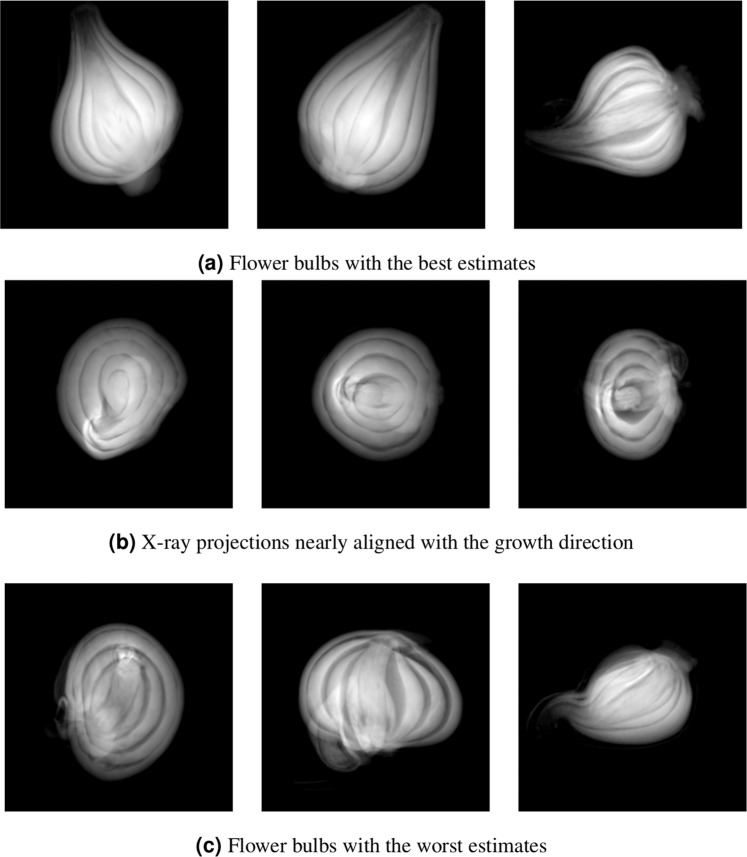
Examples of x-ray projection images from flower bulbs with both (a) good and (b,c) bad growth direction predictions from our framework. Note that the framework performs well when the bulbs are reasonably symmetric with clear shells and a noticeable tip. Worse results are seen when the bulbs have a curved stem, low contrast between shells, or have growths on the outside of the bulbs. Additionally, x-ray projection images where the normal of the projection plane is nearly in line with the growth direction of the flower bulb also proved challenging for the framework. See text for further details.
